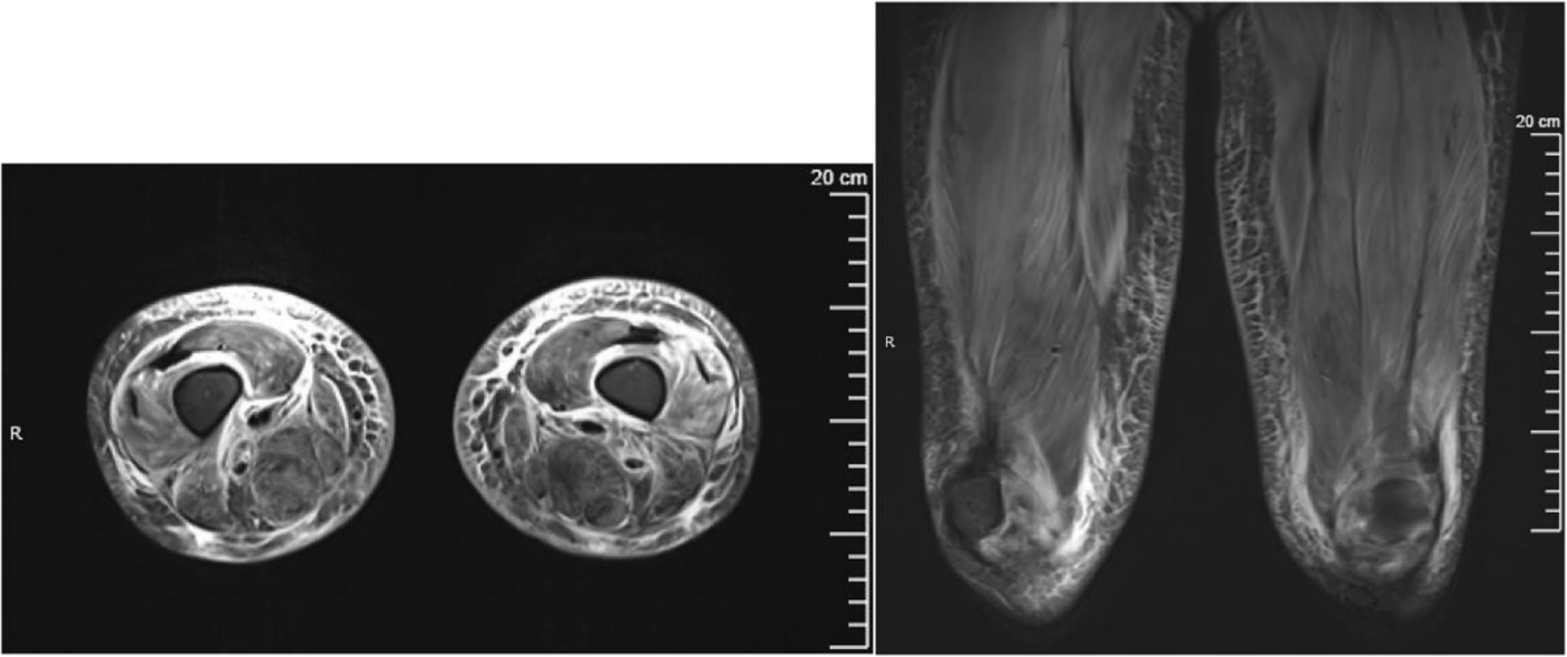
MRI Case 1: Axial and coronal STIR sequences demonstrating diffuse bilateral muscle edema, fasciitis, and subcutaneous edema
